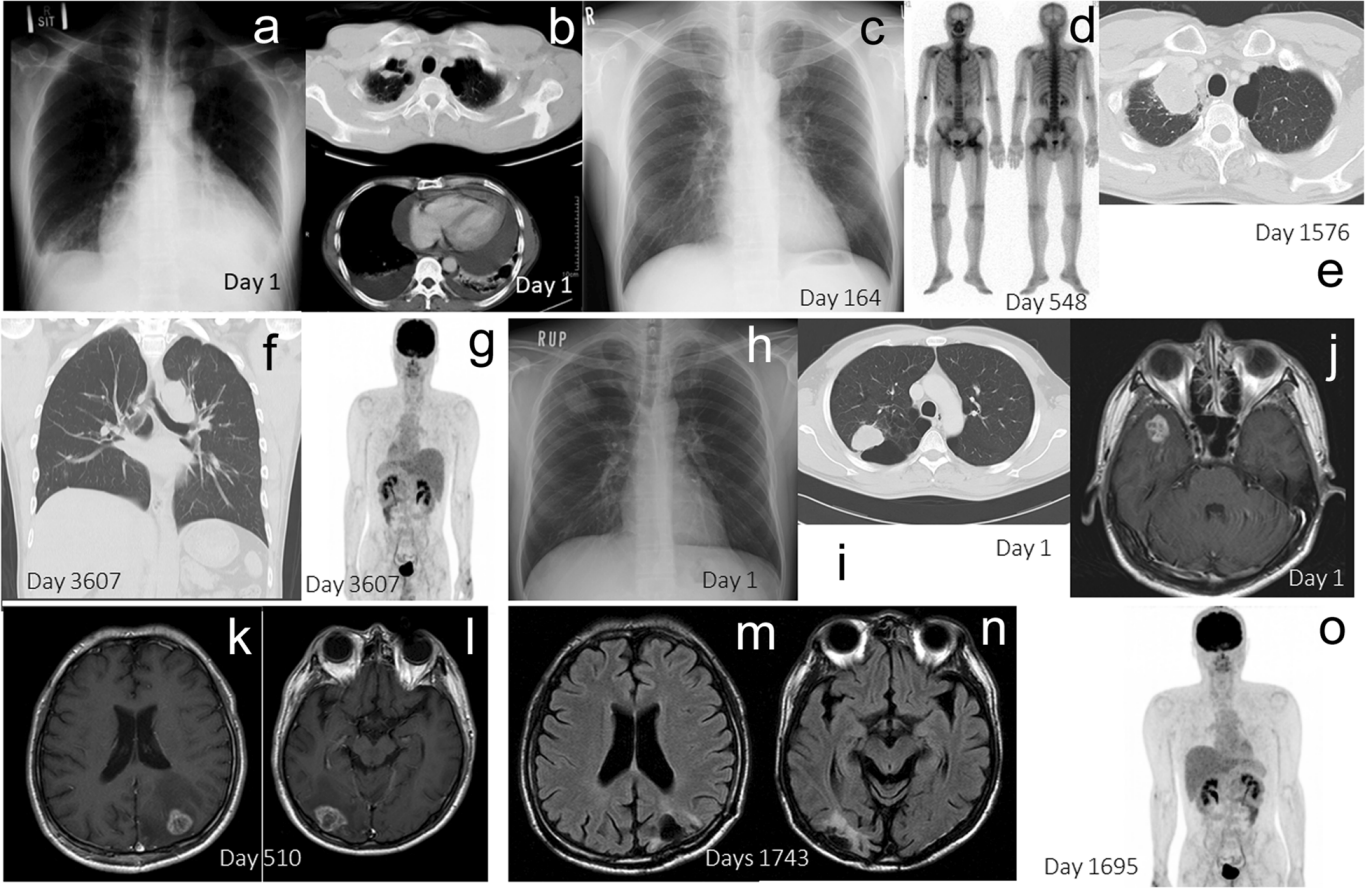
Two representative complete remission (CR) patients with oligometastases in stage IV. The first CR patient in stage IV is shown in a-g. A male in his 50s presented with dyspnea, and bilateral malignant pleural and pericardial effusions were observed on the chest X-ray (a) and the CT (b). He was diagnosed as stage IVA (cT1aN2M1a) adenocarcinoma. After pericardial drainage followed by chemotherapy, the pleural and pericardial effusions disappeared (c). He complained of left femoral pain, and bone scintigraphy revealed bone metastases in the left femoral bone and left hip joint (d). After radiation therapy, he underwent joint replacement surgery. The primary lesion, located in the right upper lobe, showed gradual enlargement (e) and was surgically removed (f). The CR has since been maintained, based on head MRI and PET/CT (g). The second patient with brain metastases achieving CR is shown in h-o. A male in his 30s consulted our hospital due to loss of consciousness. A tumor was detected in the right upper lobe (h, i). There was no lymph node swelling, while a solitary brain metastasis was observed in the right temporal lobe (j). He was diagnosed as stage IVA (cT2aN0M1b) large cell carcinoma. Cyber-knife therapy for this lesion and surgery for the primary lesion were performed. After 4 courses of a first-line chemotherapy, three new brain metastases were detected in the left cerebellum, the right occipital lobe, and the left parietal lobe by head MRI. Cyber-knife treatments for these lesions were done. He subsequently complained of headache. Brain metastases in the left occipital lobe (k), the left cerebellum, and the right occipital lobe (l) were demonstrated by head MRI. Brain surgeries were performed for these lesions. The brain tumors and the lung tumor ultimately disappeared, as shown by the head MRI (m, n) and the PET/CT (o)
